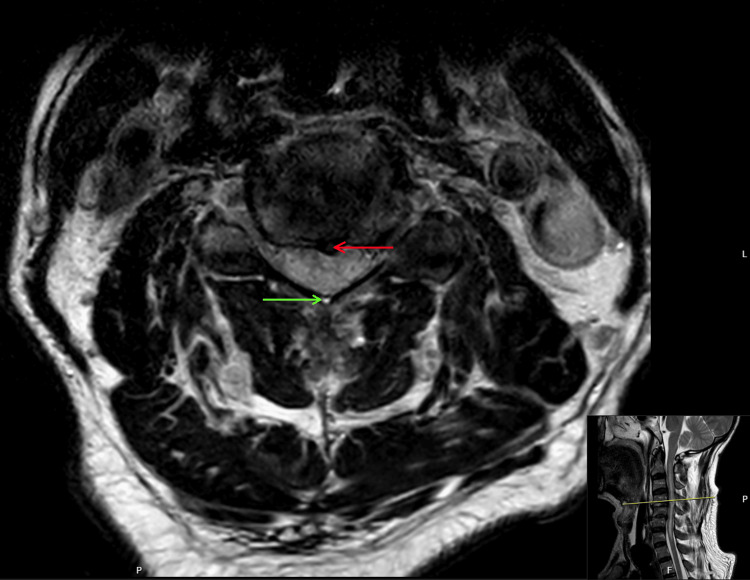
Axial T2-weighted MRI of the cervical spine at the C3 vertebral level An axial cross-section obtained at the level of the C3 vertebral body demonstrates severe mechanical compromise and narrowing of the spinal canal. The green arrow identifies a prominent focus of intramedullary T2-hyperintensity within the substance of the spinal cord, representing acute traumatic contusion and associated vasogenic edema. The spinal cord is visibly deformed and compressed into a characteristic triangular or flattened morphology: a result of the pincer effect between anterior spondylotic osteophytes and the posterior ligamentous complex. This view confirms the localised nature of the neural injury and the significant reduction in the effective canal diameter at this level. This morphology carries a poor functional prognosis MRI: magnetic resonance imaging
